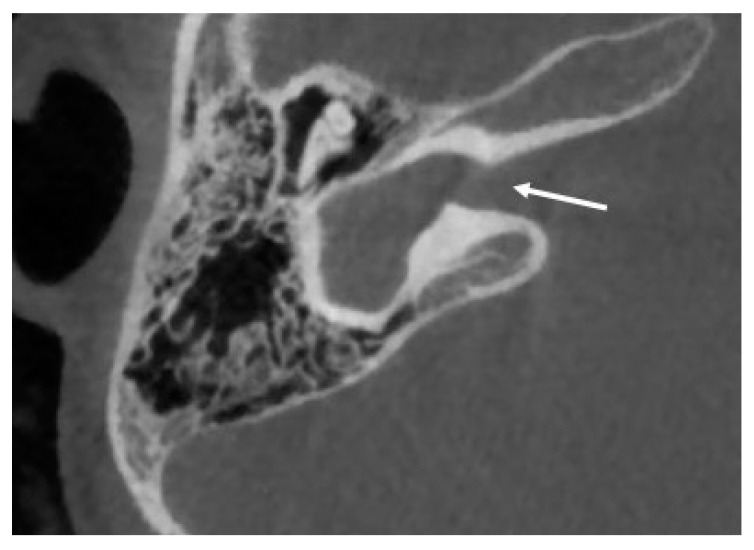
CT imaging of a cochlear aplasia with dilated vestibule. In contrast with a common cavity, the whole cochleovestibular structure is behind the axis of the internal auditory canal (white arrow).
